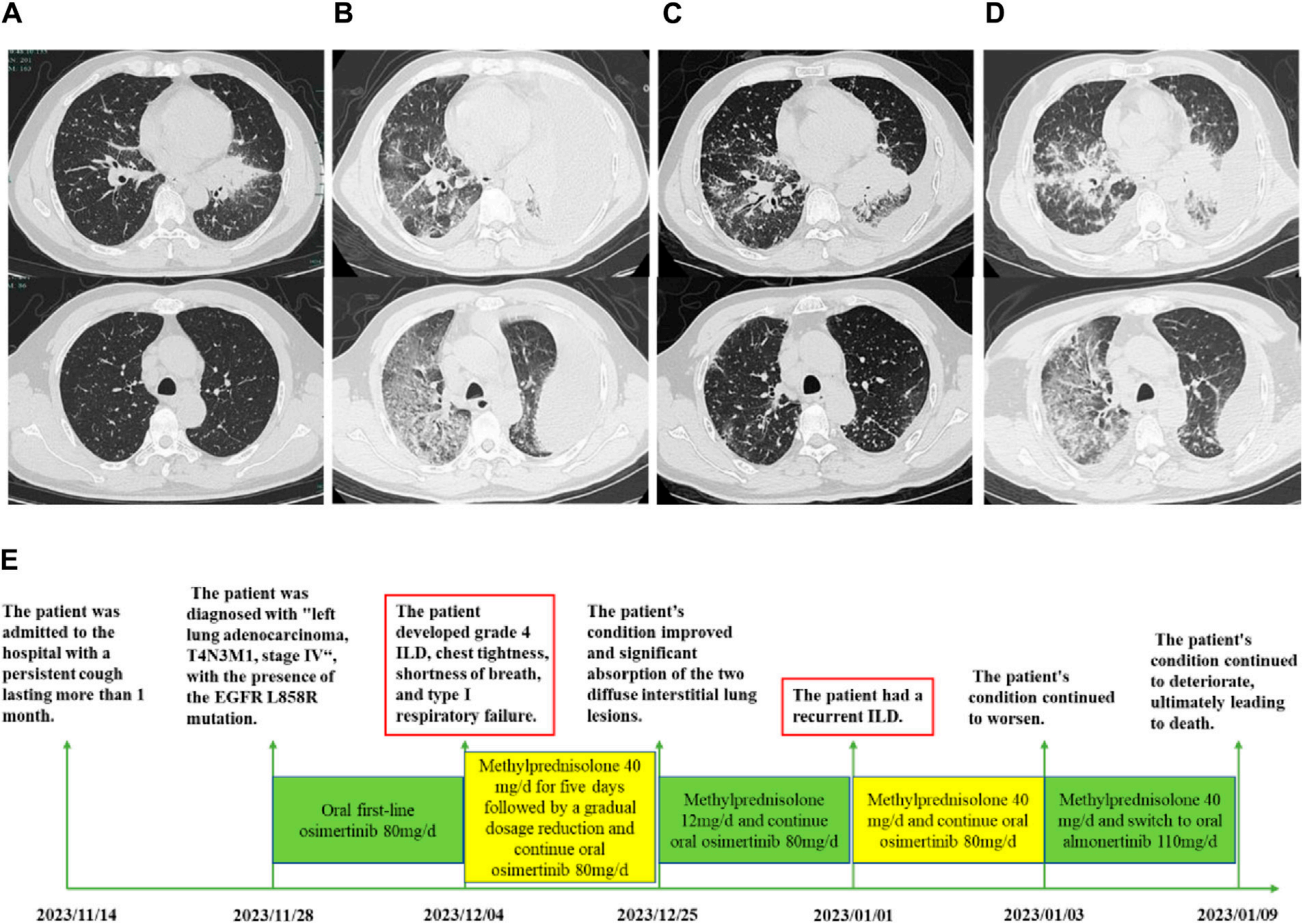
Fi (A–D) The clinical course according to CT scan findings. (A) The baseline CT scan revealed a mass in the basal segment of the lower lobe of the left lung, with no signs of pre-existing interstitial pneumonia before osimertinib therapy. (B) After 6 days of treatment with osimertinib, chest CT showed diffuse ground-glass opacities in both lungs, with the right side being more pronounced and a significant pleural effusion on the left side. (C) Following 7 days of methylprednisolone treatment, a repeat chest CT demonstrated significant resorption of the diffuse interstitial lesion identified in the previous scan, indicating a positive response to therapy. (D) On 1 January 2024, a follow-up chest CT scan displayed new diffuse ground-glass opacities in both lungs, particularly pronounced in the right lung. (E) A thorough timeline of the patient’s clinical progression and treatment protocol.
